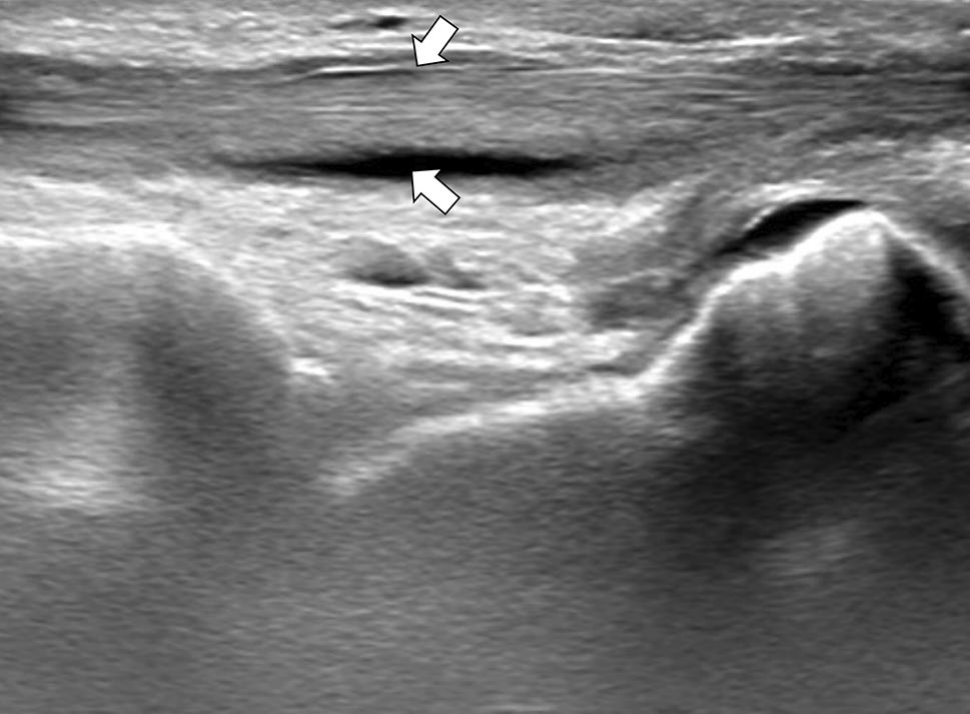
Thickening of tenosynovium, effusion, and synovial hypertrophy revealed in ultrasonography of the left musculus extensor digitorum longus tendon in long axis projection
